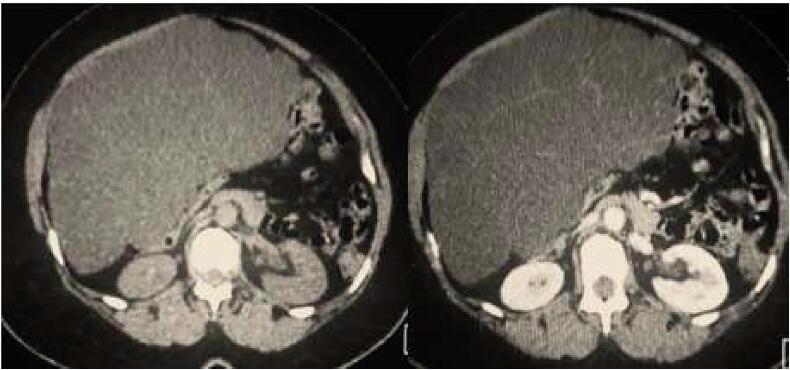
CT scan before and after contrast fluid injection: Multicystic peritoneal neoplasm displacing neighboring organs. Presence of thin walls slightly highlighted after contrast fluid injection.
